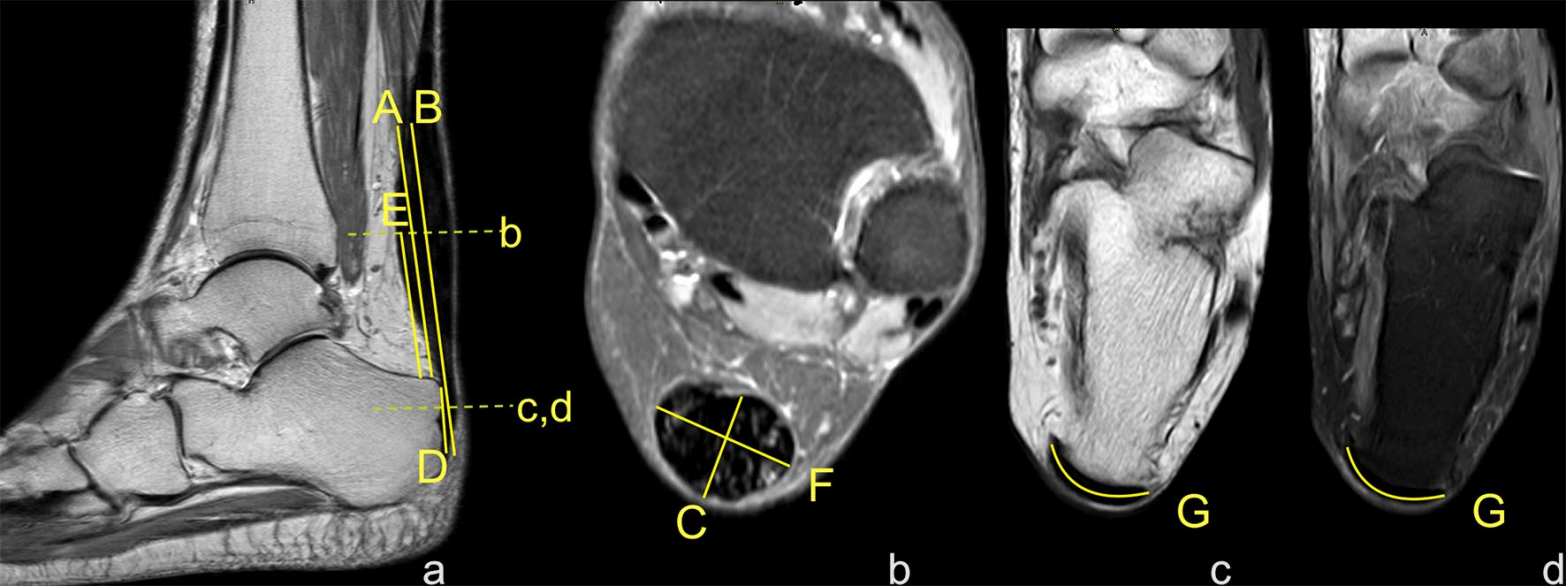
Diameters of the Achilles tendon with midportion tendinopathy of the left Achilles tendon in a 52-year-old male who presented with Achilles pain. Clinical suspicion of tendinopathy with partial rupture. MRI showed an advanced tendinopathy. A, B, C, D, F, G—dimensions of the Achilles tendon; definitions in the Material and Methods. (a) sagittal section PD- weighted, (b) PD- axial section weighted FS, (c) axial section T1- weighted, (d) axial section PD- weighted FS.
